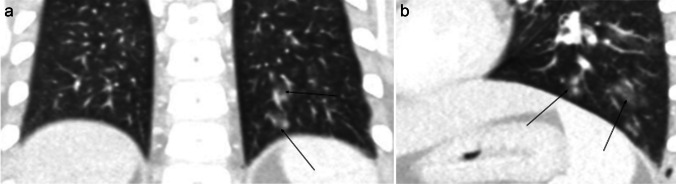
Coronal (a) and sagittal (b) contrast-enhanced lung window computed tomography images in a 7-year-old girl with coronavirus disease 2019 show small ground-glass opacities (*arrows*) in the basal and posterior lung segments – these findings were not visible on radiography
